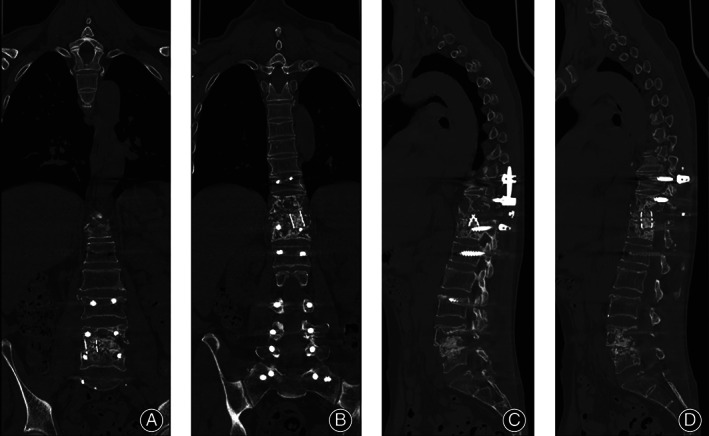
Intervertebral bony fusion was observed on CT scan at the 7‐month follow‐up.
